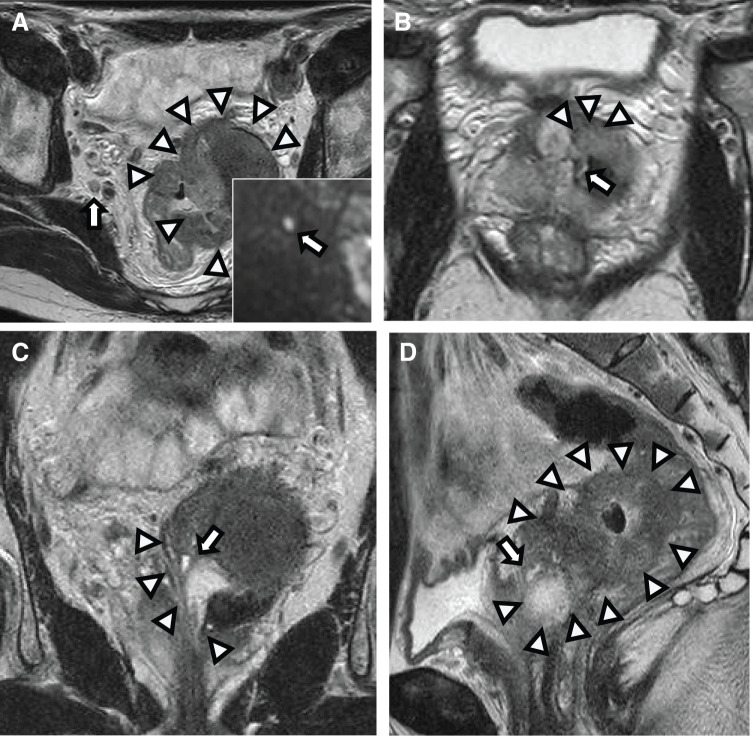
MRI findings. (A) T2-wighted, horizontal image. There is a lobulation-like tumor on the left pelvic cavity with hypointense inside. The right-sided lateral lymph node also shows hypointense (arrowheads). The lower right image indicates a diffusion-weighted, horizontal image. The right-sided lateral lymph node also shows hyperintense (arrow). (B) T2-wighted, horizontal image. The tumor connects to the prostate, invading the inner gland beyond the outer lesion (arrowheads). Arrow may indicate fistulation. (C) T2-wighted, coronal image. The tumor connects to the urinary system (arrowheads). Arrow may indicate fistulation. (D) T2-wighted, sagittal image. The tumor widely spreads and connects to the prostate, indicating direct invasion (arrowheads). Arrow may indicate fistulation.
